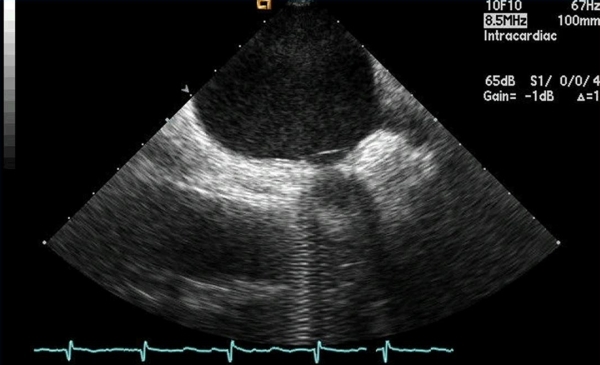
Intracardiac ultrasound image showing the circumferential mapping catheter (arrow) exactly at the ostium of the pulmonary vein.  Especially for the left-sided veins, ultrasound imaging can be helpful in approximating where the pulmonary vein ostium is located.  LA - left atrium
